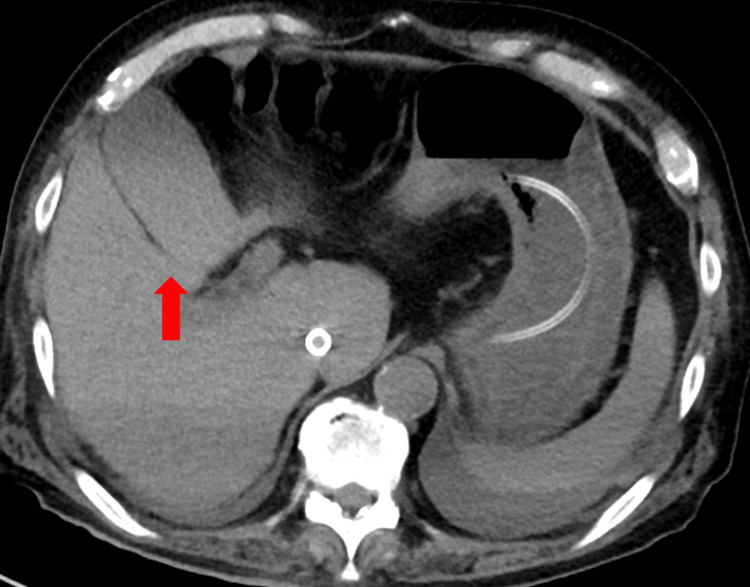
Computed tomography of the gallbladder on the twenty-seventh day Enlargement of the gallbladder is evident, with a pale hyperabsorptive zone indicating potential bile concentration or the presence of bile sludge (arrow).
